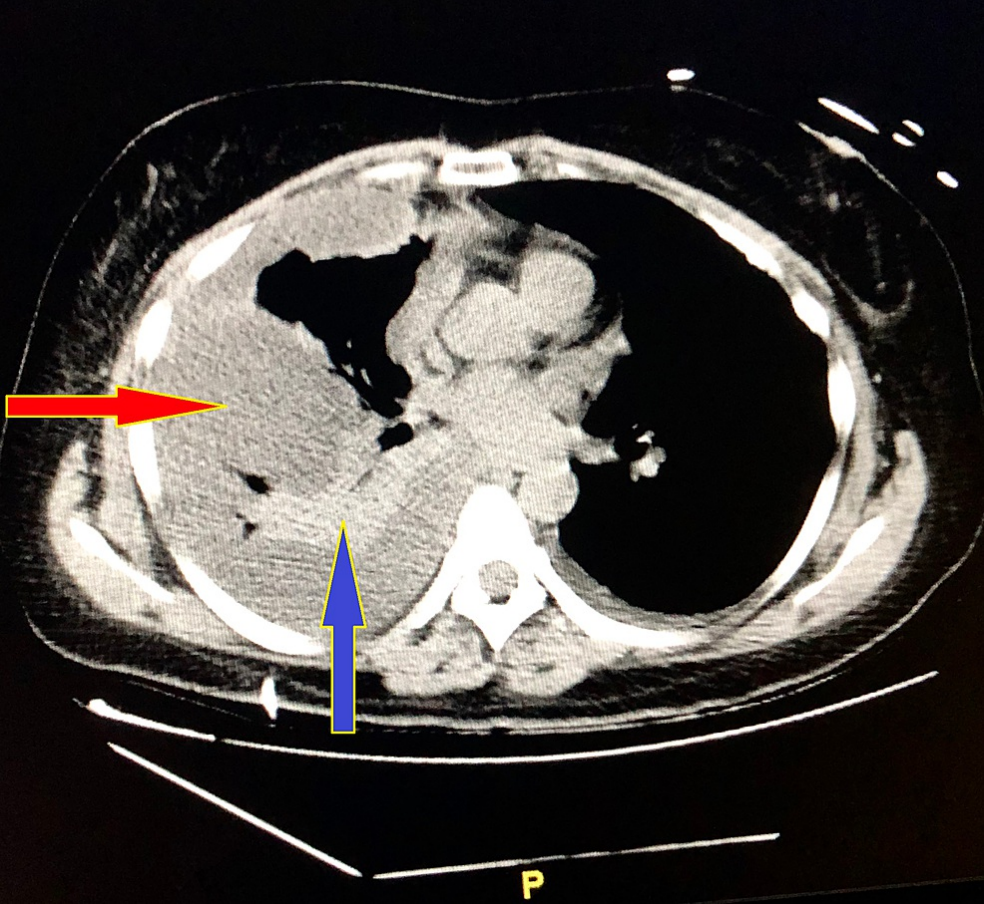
CT chest without contrast showing large right-sided encysted pleural effusion (red arrow) with collapsed lung (blue arrow)
